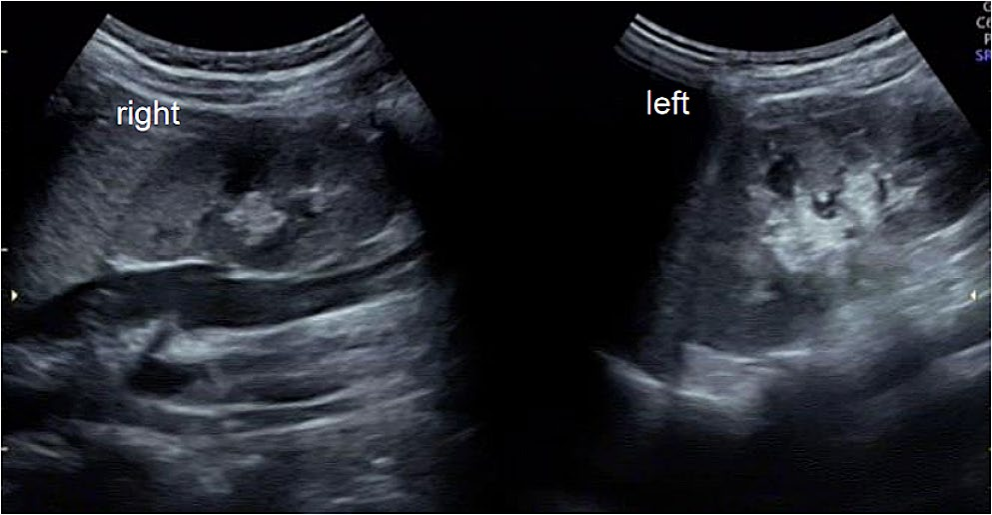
Renal ultrasound suggested bilateral normal kidneys
